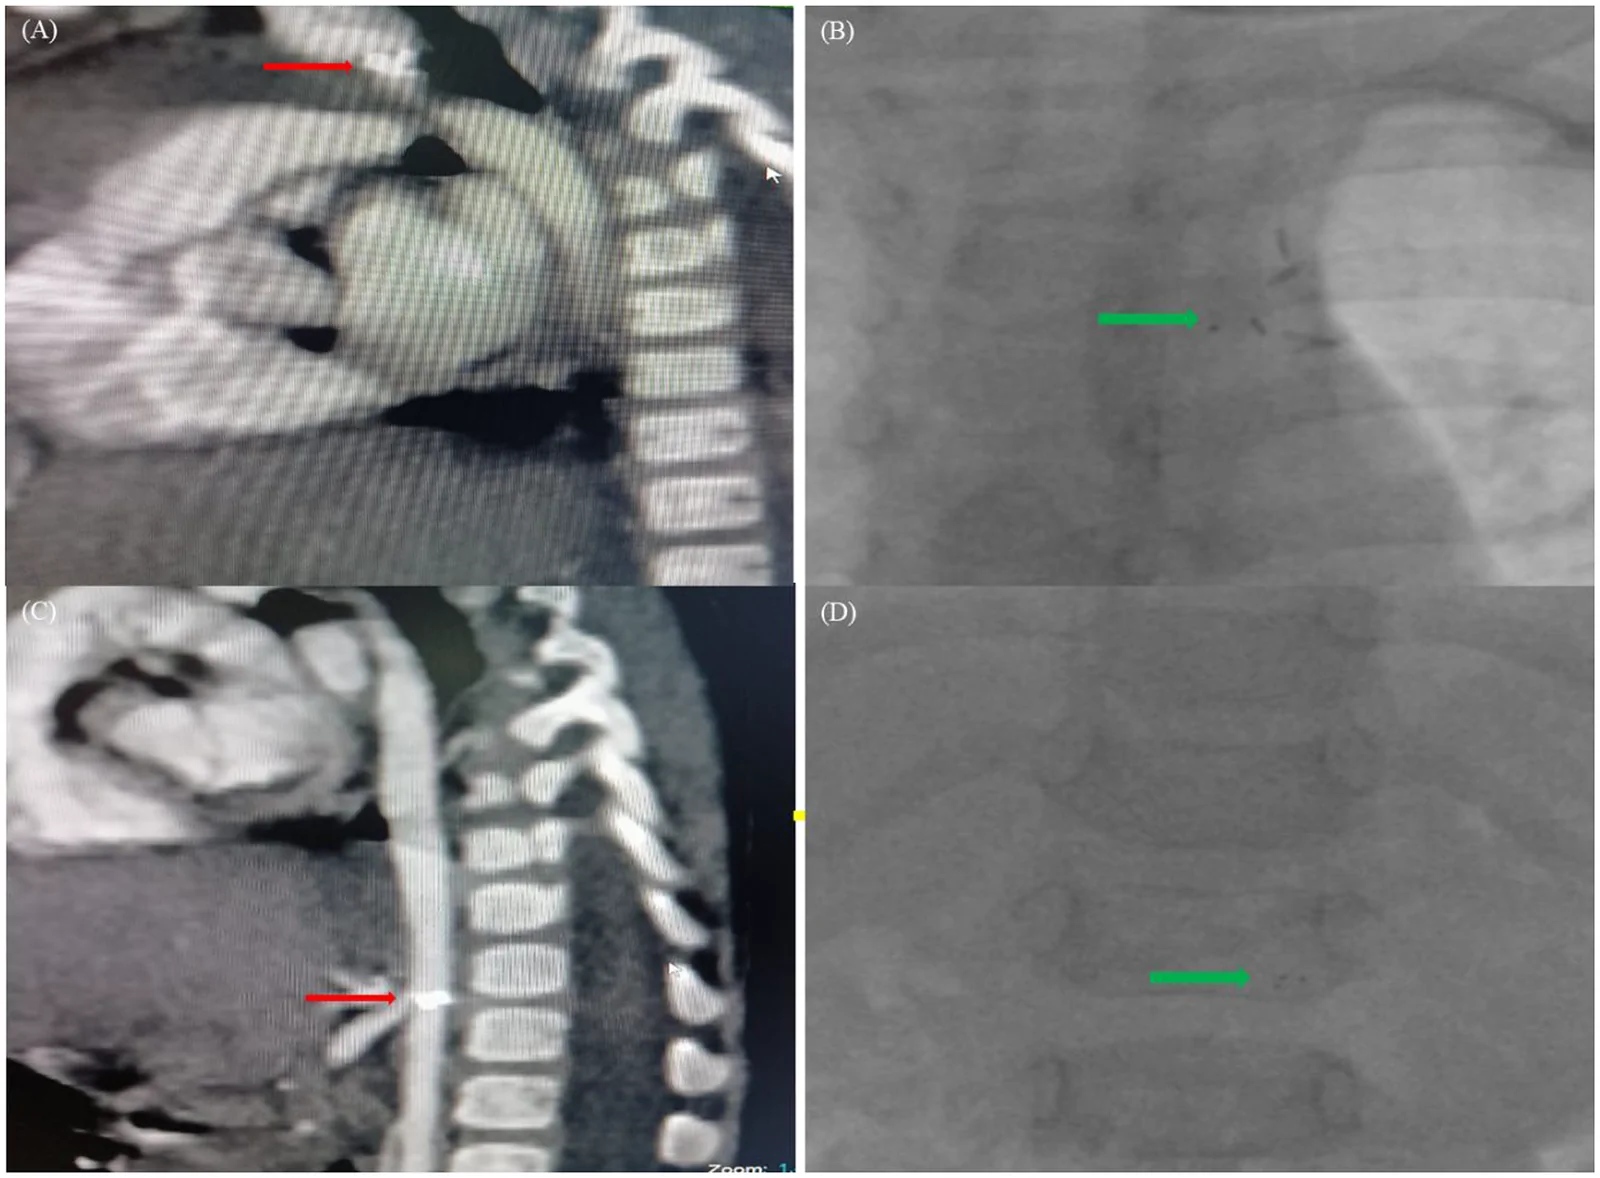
(A) CT angiogram, sagittal reconstruction, showing proximal BVS fragment near the isthmus (red arrow). (B) Fluoroscopic AP view showing proximal stent markers (green arrow) near the isthmus (fluro taken after surgery). (C) CT angiogram, sagittal reconstruction, showing distal BVS fragment in the proximal abdominal aorta (red arrow). (D) Fluoroscopic AP view showing distal stent markers in the proximal abdominal aorta
